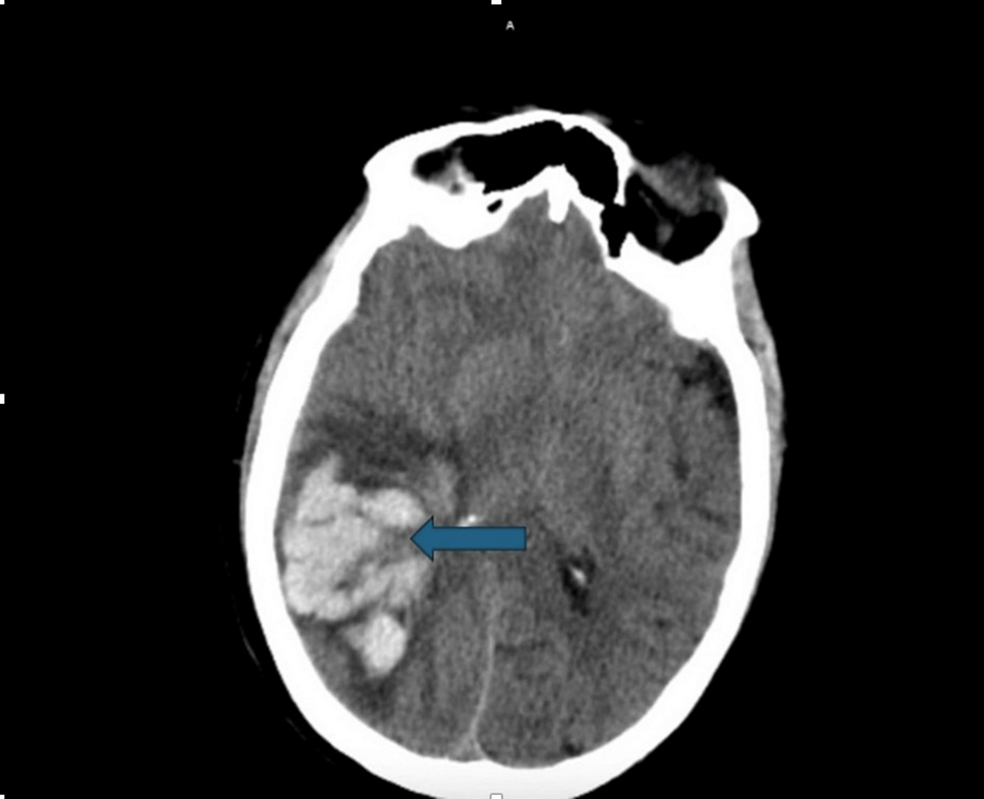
A CT scan of the head shows a large intraparenchymal hemorrhage measuring 6.8 x 4.8 x 7.4 cm.
